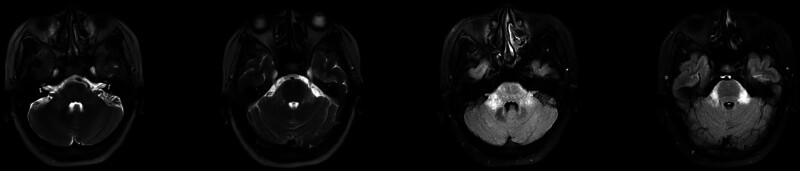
The brain MRI showed abnormal hyperintense signals with diffused borders in the bilateral pontine brachium, predominantly on the right side.
